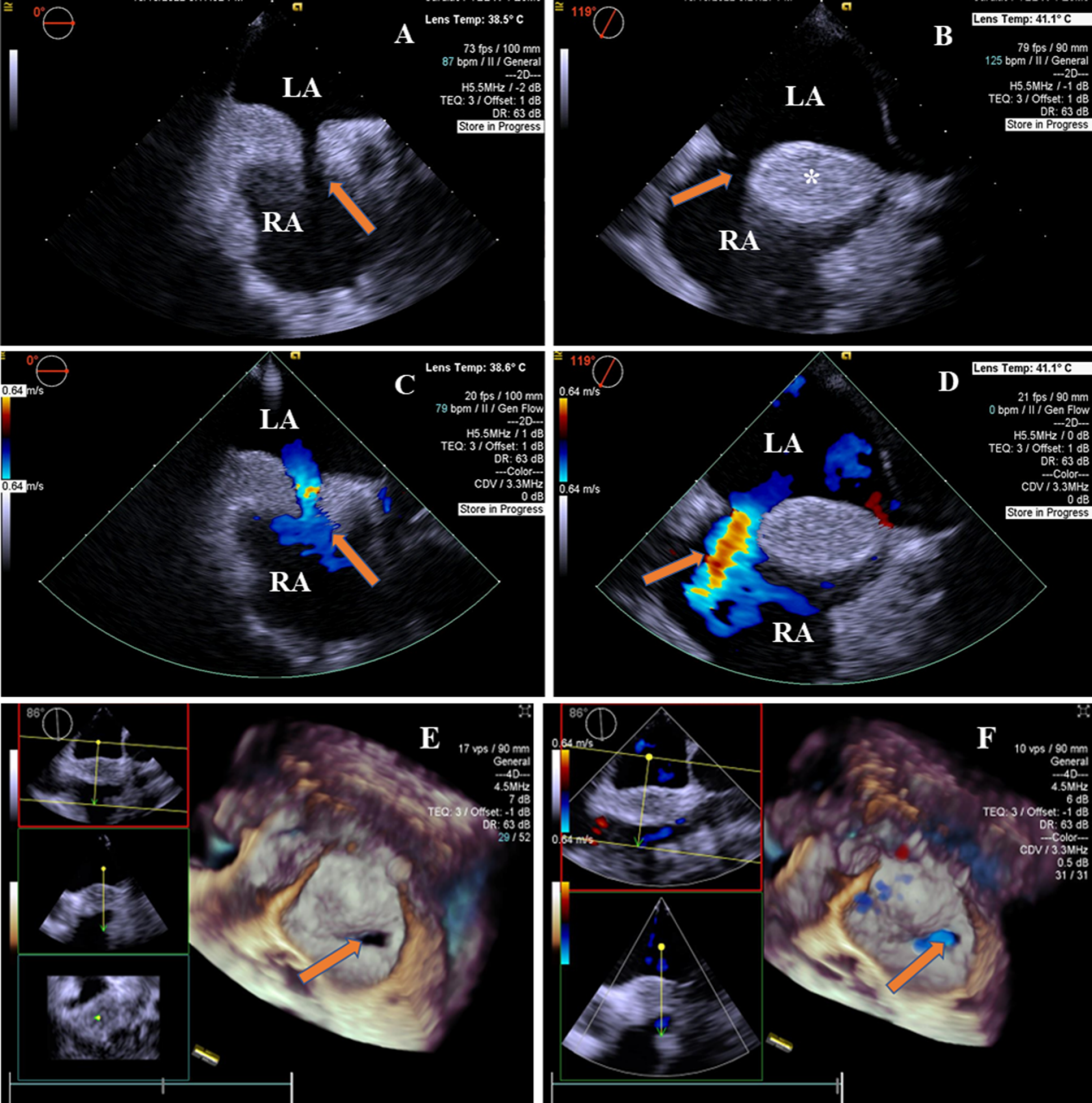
2D-TEE and 4D-TEE images revealed lipomatous hypertrophy of the atrial septum with atrial septal defect. (A) On 2D-TEE imaging, a single secundum ASD (arrow) and interatrial septum thickened to 20.7 mm in diameter were observed from 0° view. The septum with brightness features is characterized by the deposition of unencapsulated fat cells in the atrial septum. (B) 2D-TEE further documented a “dumbbell”-shaped involvement of the cephalad and caudal regions with sparing of the fossa ovalis (arrow) from 119° view. The interatrial septal fatty infiltration was demonstrated (*). (C) Color Doppler ultrasound of 2D-TEE showing a blood shunt from the left atrium to the right atrium (arrow) from 0° view. (D) Color Doppler ultrasound of 2D-TEE showing left-to-right shunt through ASD (arrow) from 119° view. (E) RT4D-TEE imaging reflected the whole ASD was surrounded by lipomatous hypertrophy of atrial septum (arrow) from 86° view. (F) Color Doppler ultrasound of RT4D-TEE showing blood flow of the ASD from the left to the right atrium (arrow). LA, left atrium; RA, right atrium.
